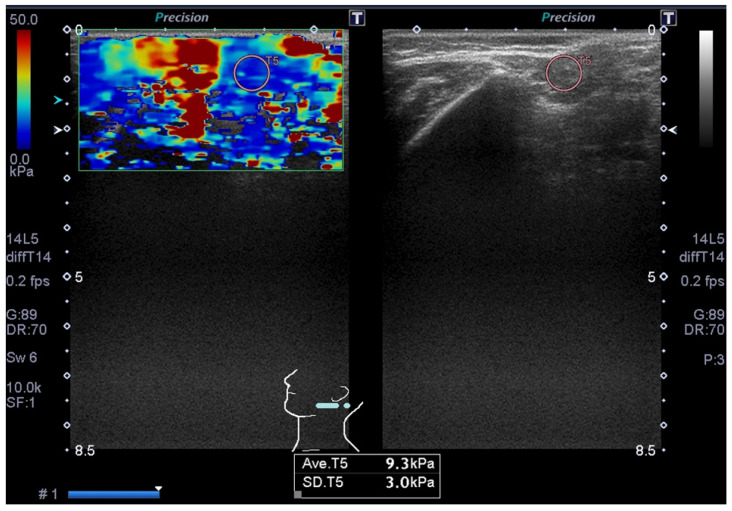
Ultrasonography of left parotid gland in a 75-year-old female with tongue carcinoma. Ultrasonography indicates heterogeneity in internal architecture of parotid gland on B-mode. Shear wave elastography indicates that shear elastic modulus of parotid gland is 9.3 ± 3.0 kPa.
